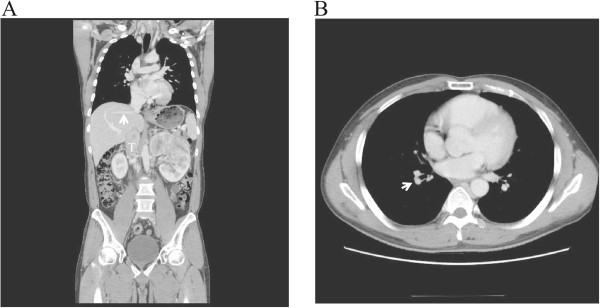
Computed tomography scan of renal cell carcinoma with tumor thrombus (level II). (A) Computed tomography scan of a 37-year-old man with left renal cell carcinoma showing a large tumor thrombus (T) extending into the retrohepatic inferior vena cava below the main hepatic vein (white arrow, level II). (B) A small embolism (T3bN0M1) of the right pulmonary artery (white arrow).
